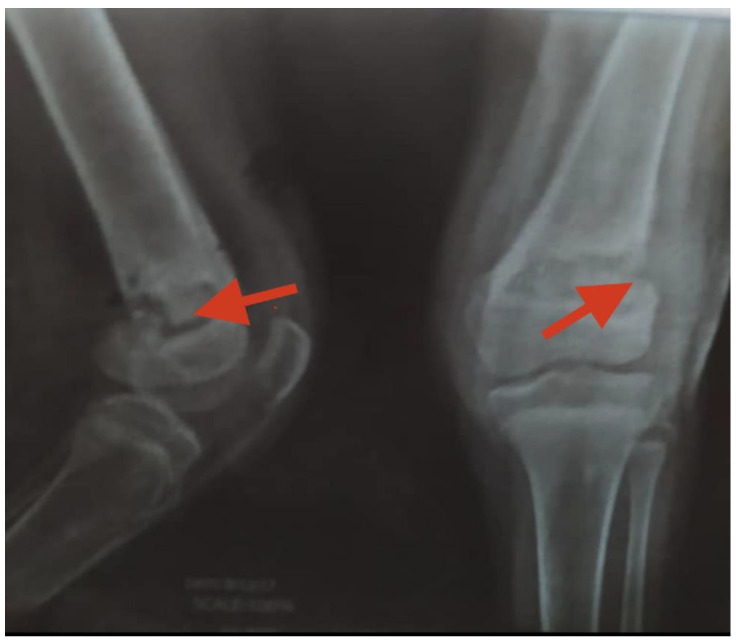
Plain radiograph of the right and left knee with osteomyelitis (red arrows) following the insertion of percutaneous cross-pins.
